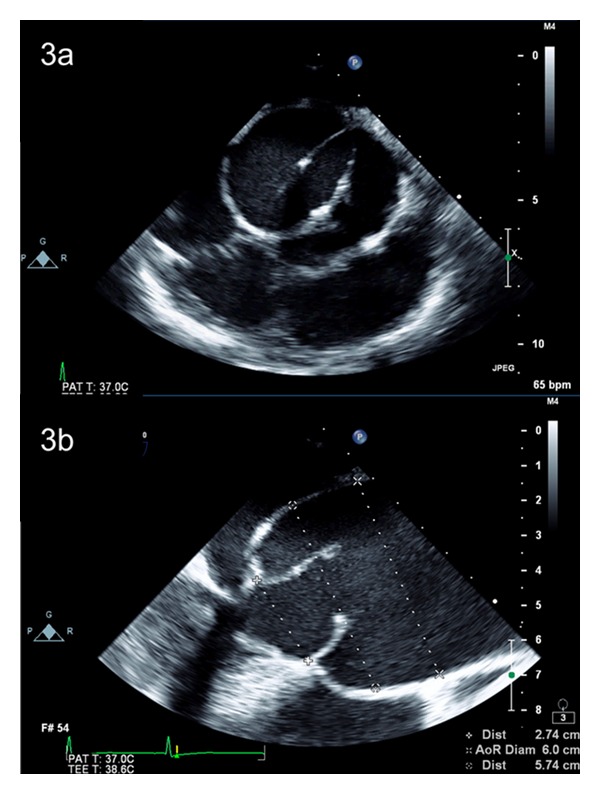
(a) Transesophageal echocardiogram demonstrating a bicuspid aortic valve in short-axis view, with left-right coronary cuspal fusion (Sievers' classification type 1, L-R, insufficient), and moderate-severe aortic insufficiency on colour flow Doppler (not shown). (b) Transesophageal echocardiogram demonstrating a dilated aortic root and ascending aorta in long-axis view along with a bicuspid aortic valve.
